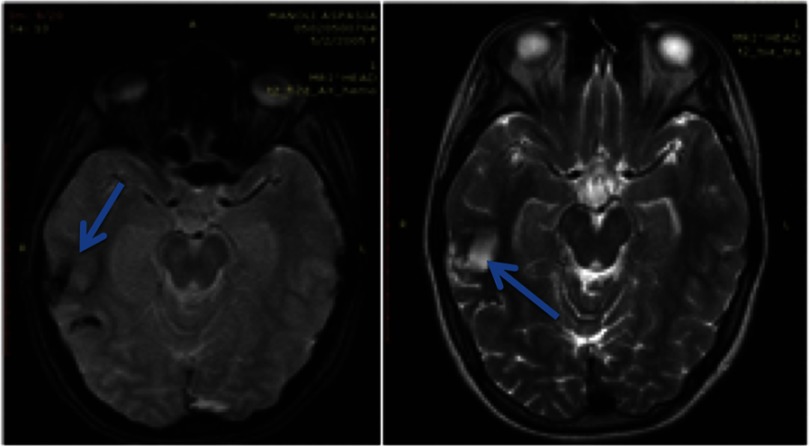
The left image is an MRI GRE sequence, showing the hemosiderin ring (arrow) and the right image is a FLAIR sequence, revealing an area of intermediate to hypointensity signal (arrow).
